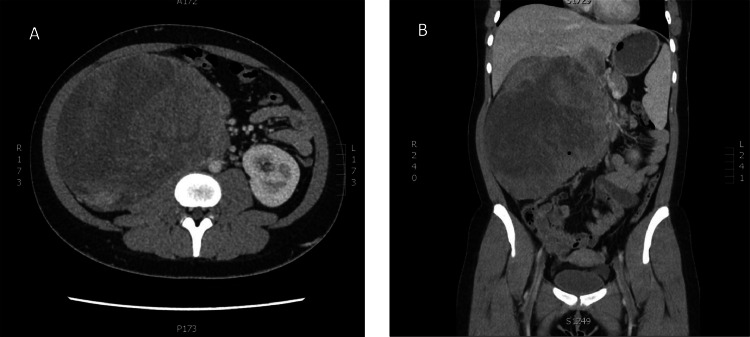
Axial CT showing (a) large tumor arising within the right retroperitoneum completely replacing the right kidney with tumor mass infiltrating the inferior vena cava. Coronal CT showing (b) the large retroperitoneal mass with thrombus extending into the proximal and distal IVC and right hepatic vein.
